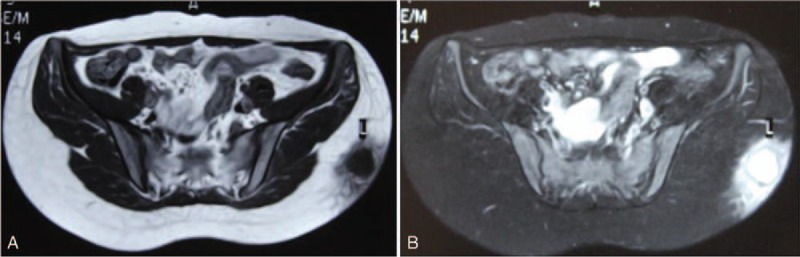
(A and B) Preoperative transverse magnetic resonance imaging scan revealing the soft tissue tumor on left buttock.
